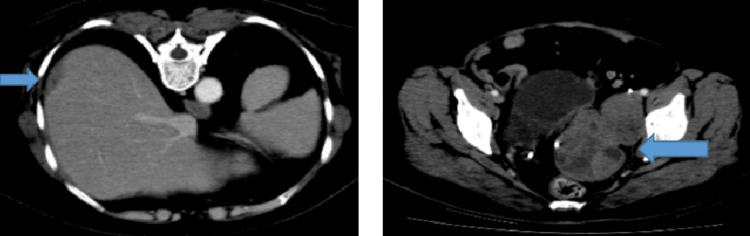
CT scan axial views showing progressive disease in terms of an increase in the size of bilateral adnexal masses and extracapsular hepatic metastasis.
